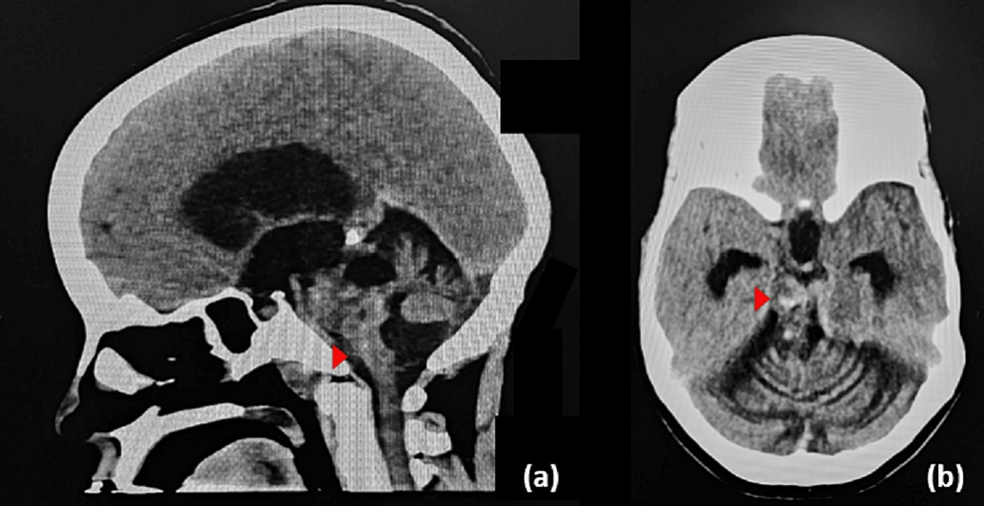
Follow-up plain computed tomography imaging The follow-up sagittal (a) and axial (b) cut of plain CT scan showing interval stability of previously noted pontine cavernous malformation.
